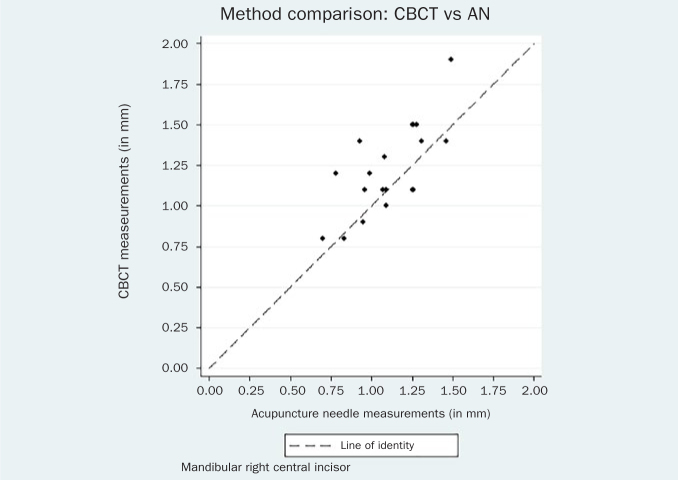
Method agreement plot CBCT vs AN 41.
